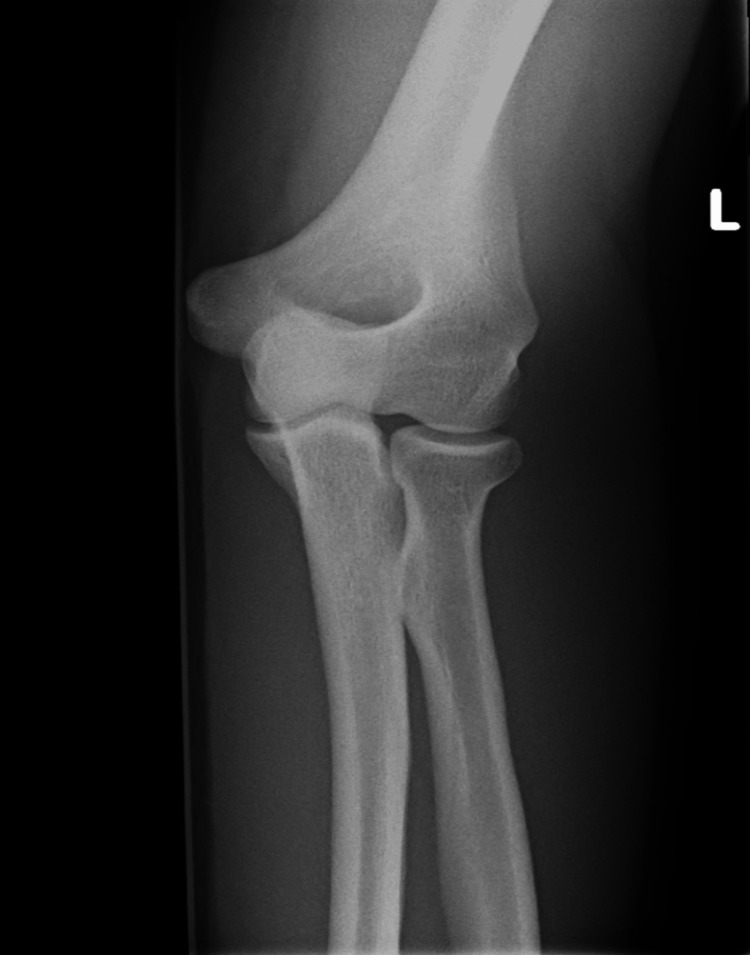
X-ray anteroposterior view of patient's left elbow Anteroposterior view of the left elbow is relatively normal with no features of osteomyelitis or bony abnormalities.
